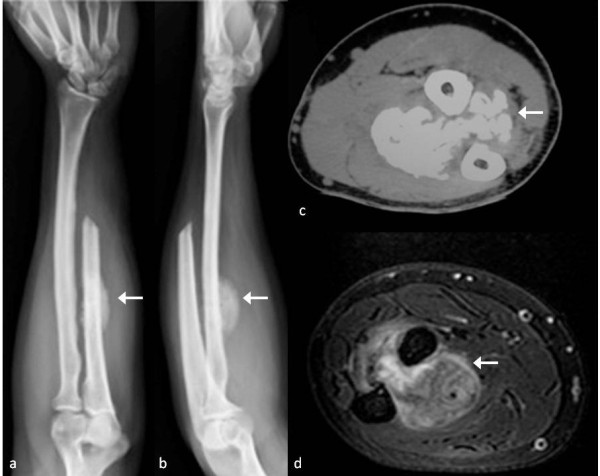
X-rays (a, b), CT scan (c) and MRI (d) show increased dimensions of the lesion but no contact with the bones after a few weeks.
